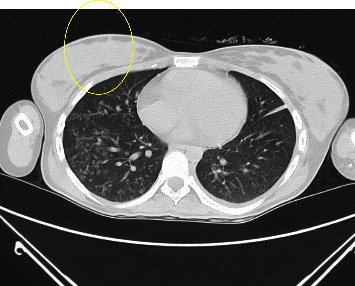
CT chest image demonstrates multiple tree-in-bud opacities within the right lower lobe (yellow circle). Findings can also be seen in the setting of aspiration or other atypical/fungal infections, sarcoidosis, and some viruses such as CMV, among other etiologies.
